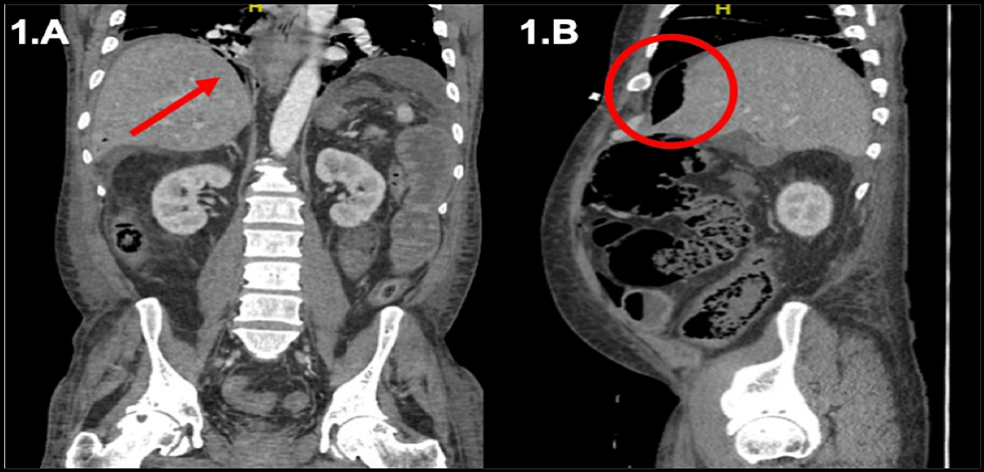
CT Abdomen with thin lucency below the right hemidiaphragm (A) and moderate pneumoperitoneum in the right upper quadrant and anterior abdomen (B) suggestive of moderate pneumoperitoneum. CT = computerized tomography
